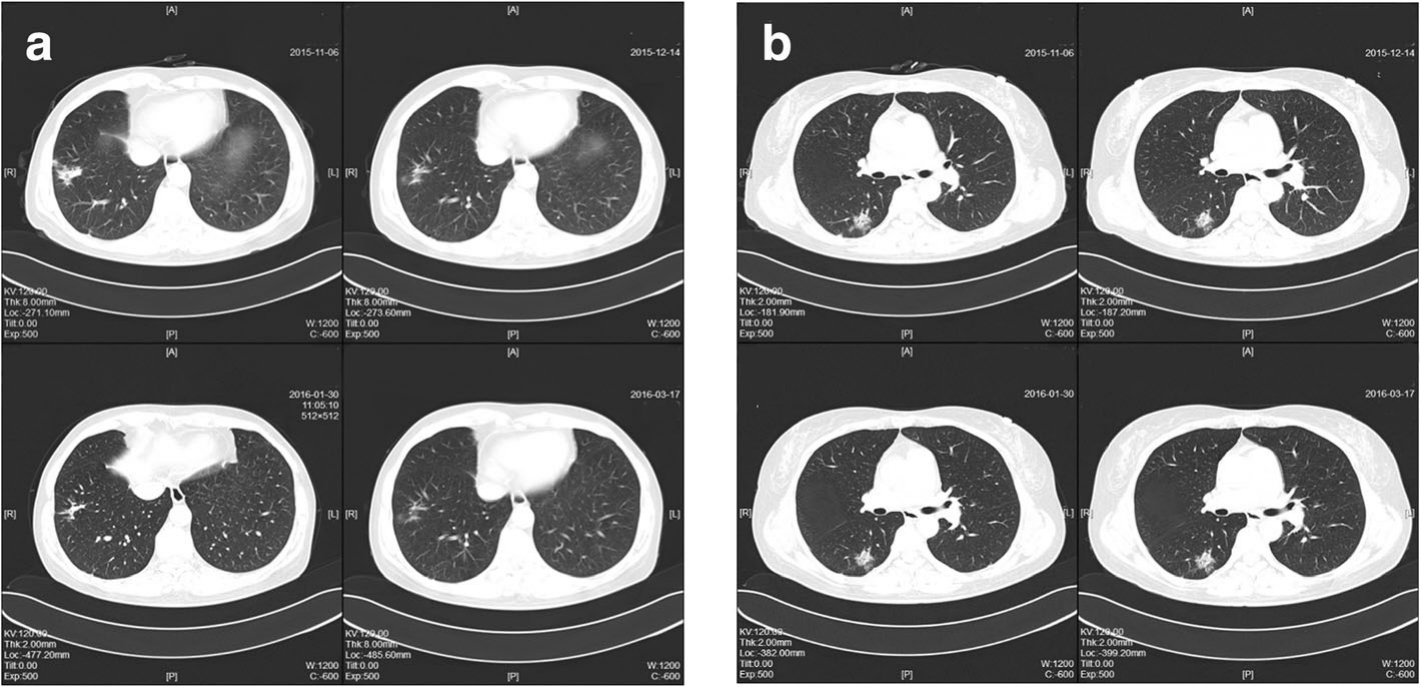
During her 6-month treatment, the patient underwent computed tomographic examination four times. a Great improvement in the patient’s lesion of the right lateral basal segment. b The nodule in the right posterior segment remained almost the same as before
